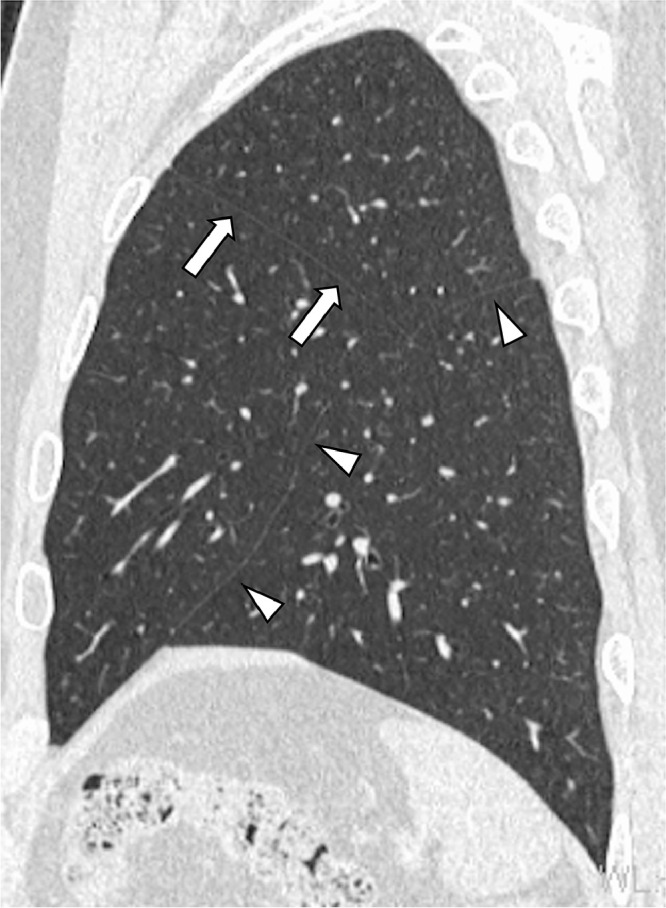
An accessory fissure (arrow) divides the left upper lobe into the lingula and the rest of the lobe on the reconstructed sagittal computed tomography image. A normal oblique fissure (arrowheads) is also present and the left lung is divided into 3 lobes.
